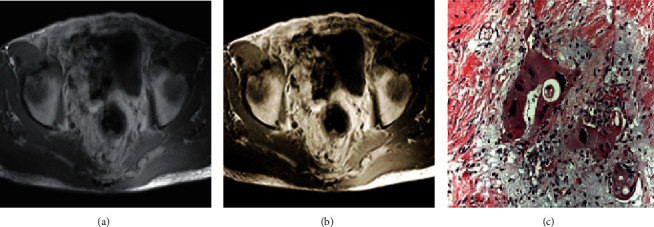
Comparison of conventional MRI, optimized MRI, and pathological results after NCRT. (a) Conventional MRI image diagnosed as TRG2; (b) optimized MRI image diagnosed as TRG2; and (c) pathological results diagnosed as TRG3.
